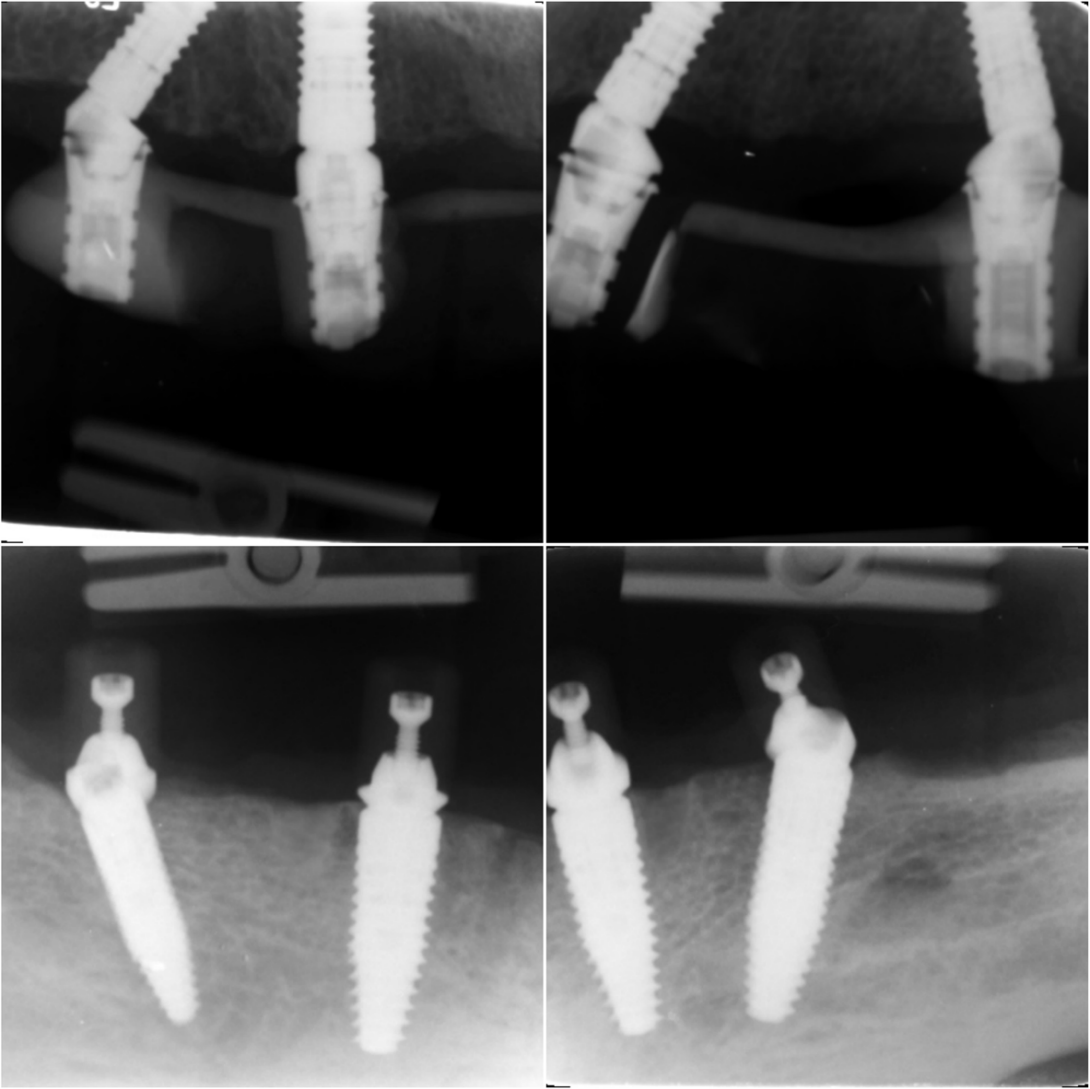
Radiological follow-up of patient was performed with paralleling X-ray
